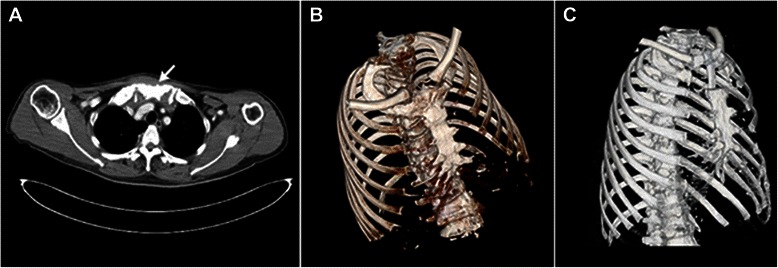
Radiologic images. Chest CT axial view (A) and computed tomographic scan of the reconstructed chest (B) before surgery showed the osteolytic lesion (arrow) in the manubrium. Computed tomographic scan of the reconstructed chest (C) was followed up 3 months postoperatively.
